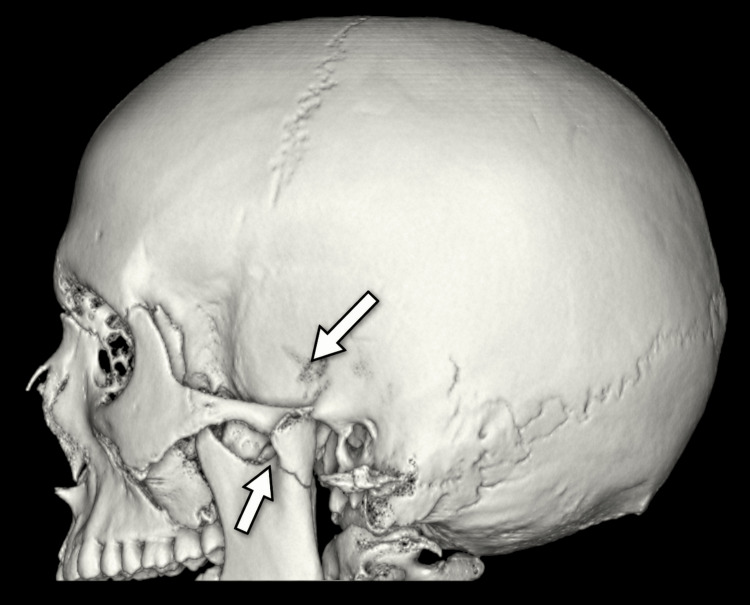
Preoperative 3D CT lateral volume-rendered image demonstrating a left-sided condylar fracture with superolateral TMJ fracture-dislocation. The upper arrow indicates the superolaterally displaced condylar fragment relative to the zygomatic arch, while the lower arrow indicates the fracture line at the condylar head/neck region. CT, computed tomography; TMJ, temporomandibular joint
